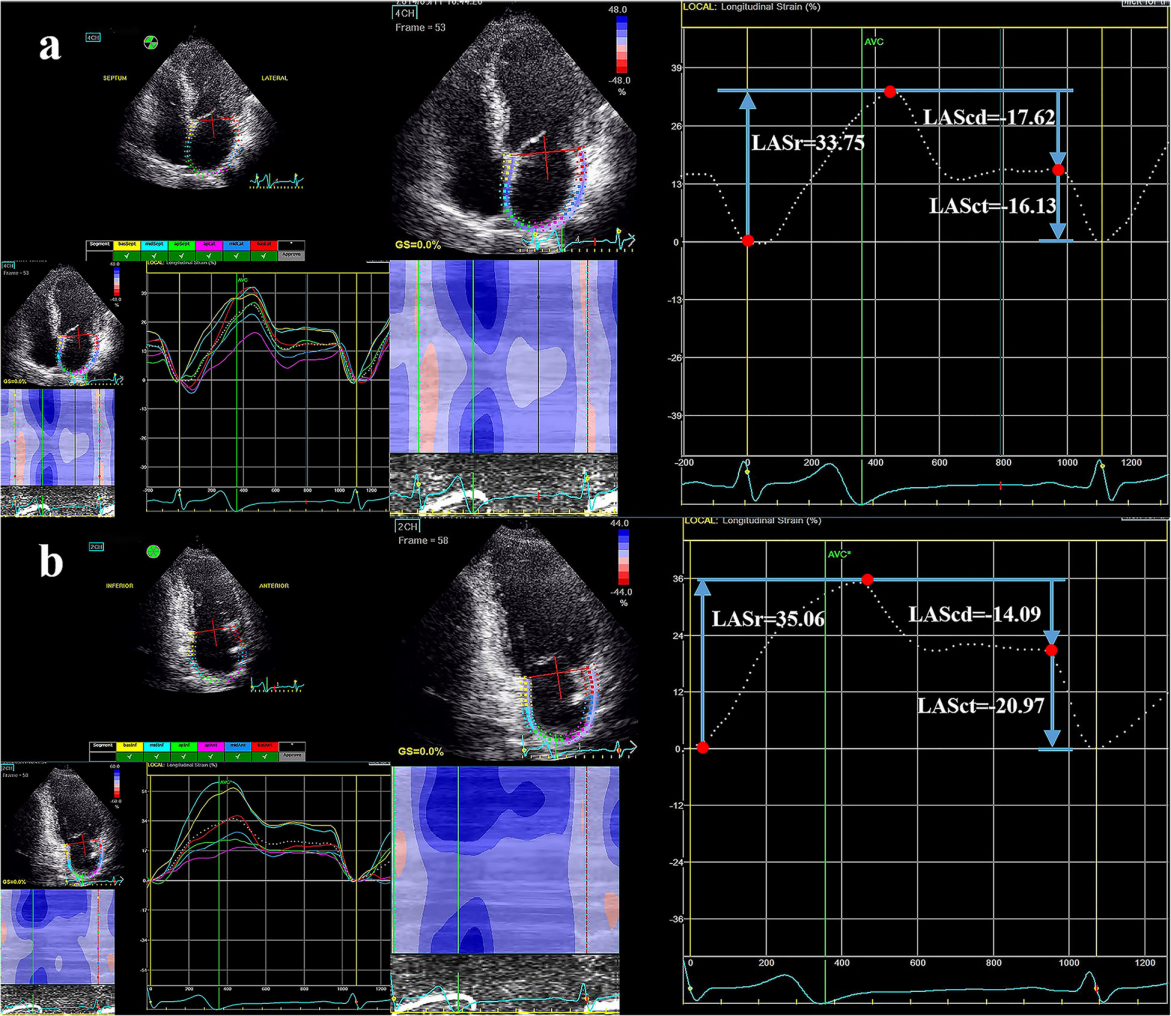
Representative example of two-dimensional STE–derived left atrial strain measurements during the reservoir, conduit, and contraction phases using an apical four-chamber view (a) and apical two-chamber view (b). Three measurement points (red dots) were used to calculate the values of deformation during the three phases. STE, speckle-tracking echocardiography
